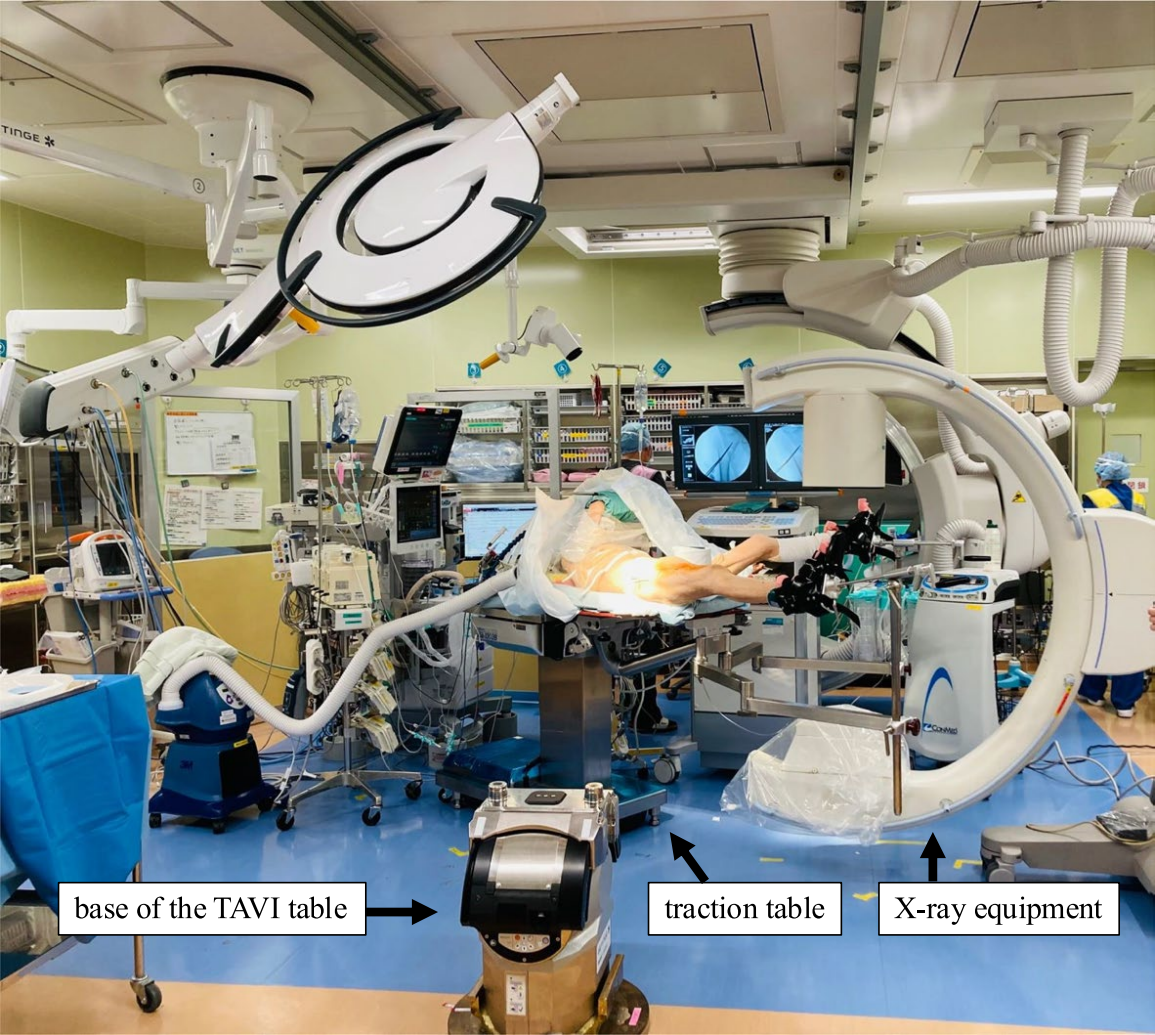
Femoral osteosynthesis in a hybrid operating room. The layout of the traction table and instruments was determined with consideration of the base of the TAVI table, the surgical lights, and the X-ray equipment
